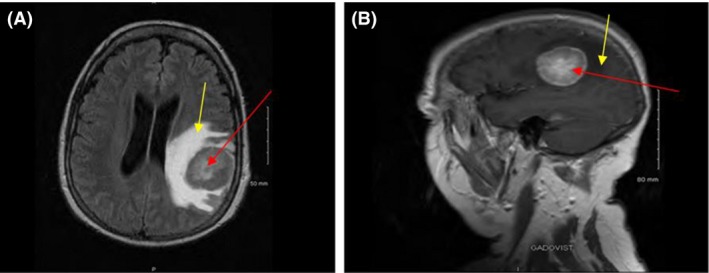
MRI brain showing a well‐circumscribed left parietal extra‐axial neoplastic mass (red arrows), with extensively associated vasogenic edema (yellow arrows) (A, axial view; B, sagittal view).
